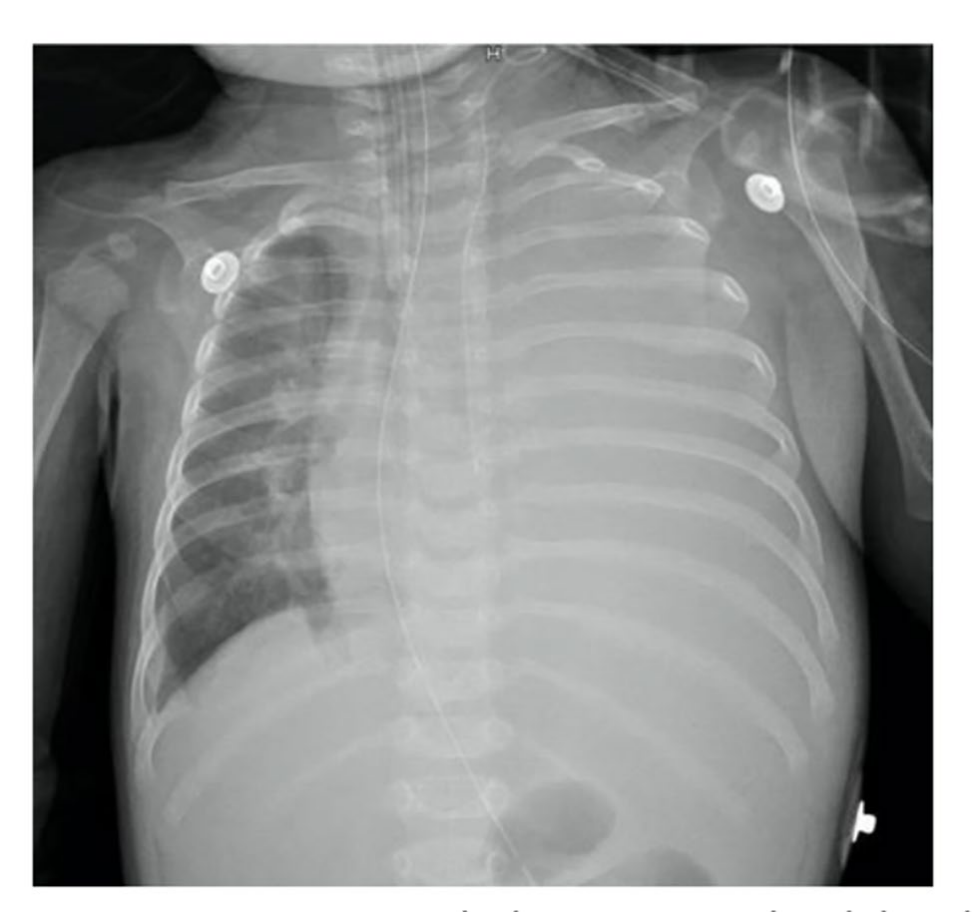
Bedside chest X-ray, engorged thorax and white lung in the left side
